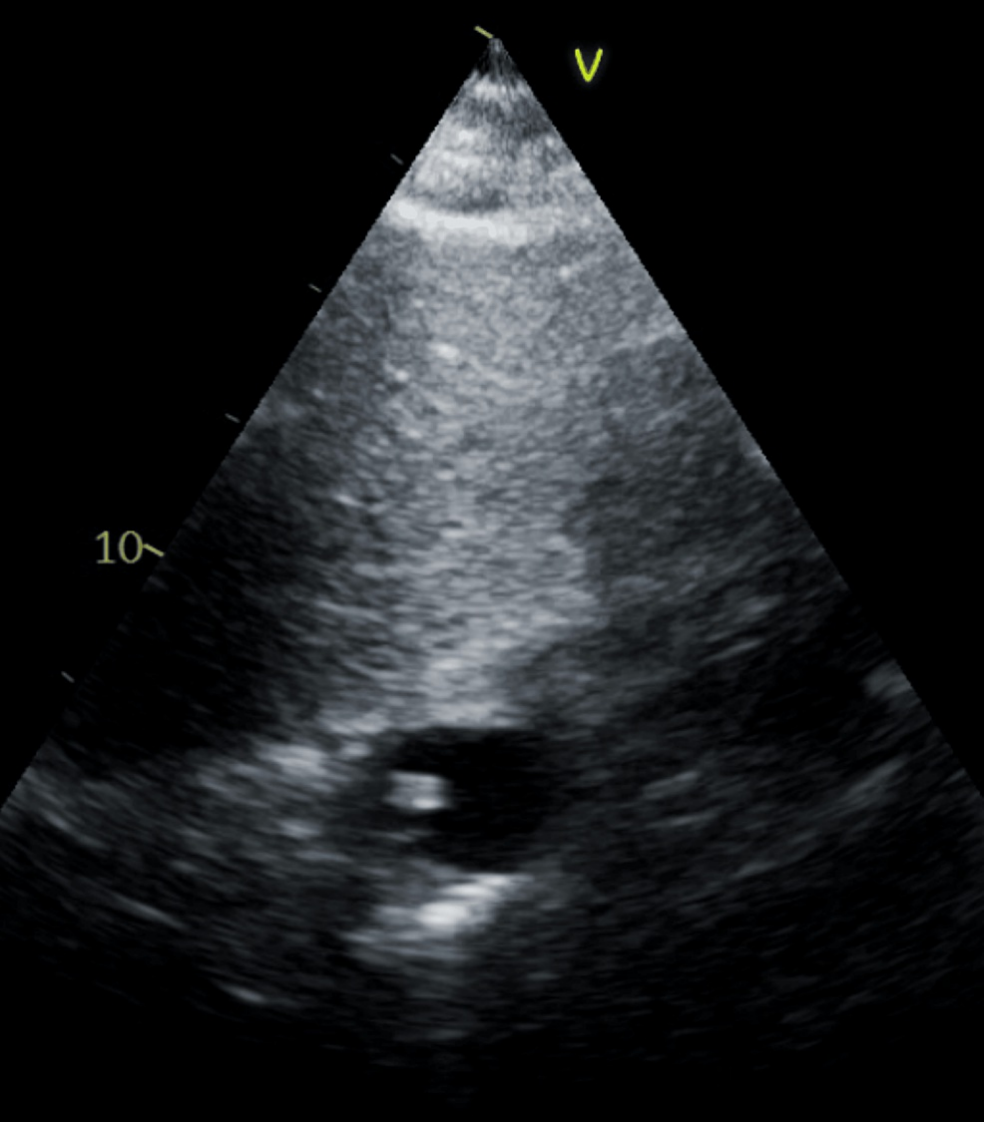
TTE subcostal view Subcostal view of the right atria with an echogenic object TTE: transthoracic echocardiogram
